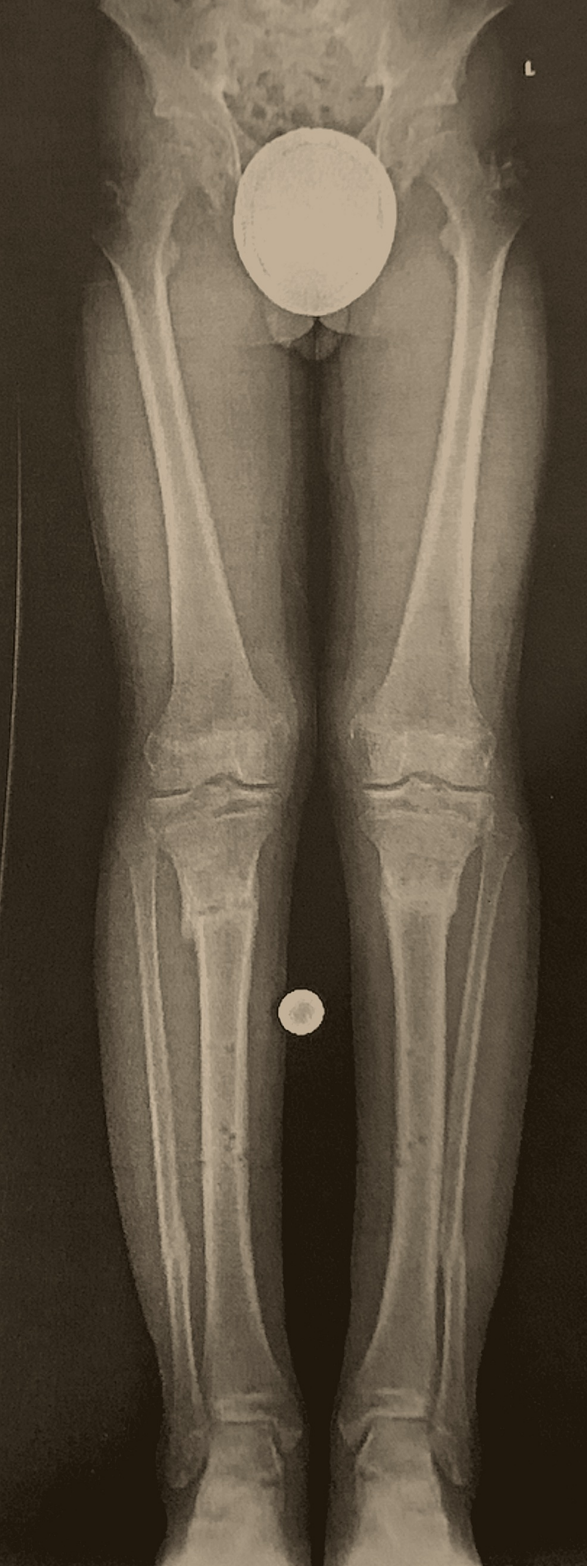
Post-operative X-ray of the lower extremity showing correction of the varus deformity
